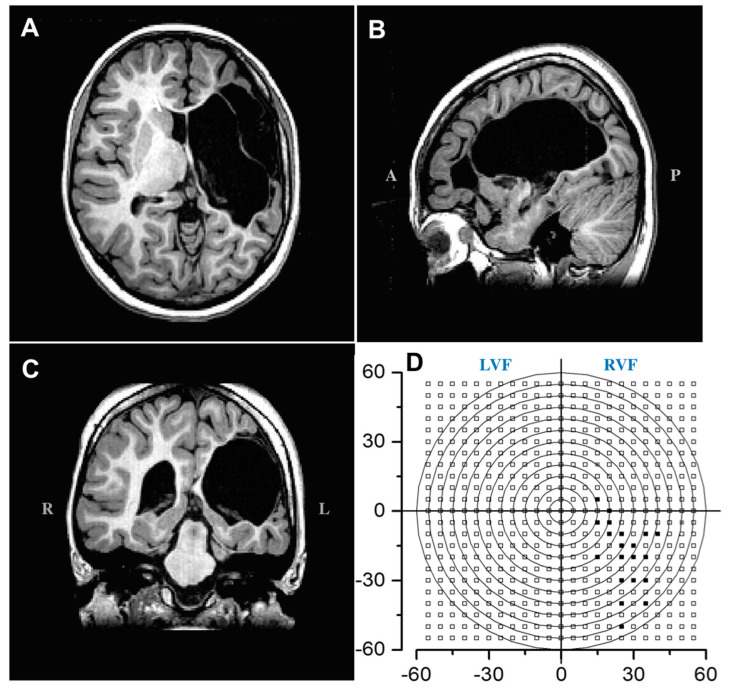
Structural T1-weighted MRI scan and visual field mapping of Patient IB. Sections showing the absence of LGN and optic radiations in the left hemisphere are displayed in (A) transverse, (B) sagittal (A-Anterior, P- Posterior), and (C) coronal planes (R- Right, L- Left). (D) Graphical reconstruction of visual field perimetry up to 60° of eccentricity. Visual field perimetry obtained with the KOWA AP 340, retaining 5° resolution of the perimetry. The contra-lateral right visual field has a very sparse scotoma in the lower field only after 15° of eccentricity. Open squares report successful detection at 15 and 17 dBs luminance levels; filled squares show an absence of detection at 0 dBs.
